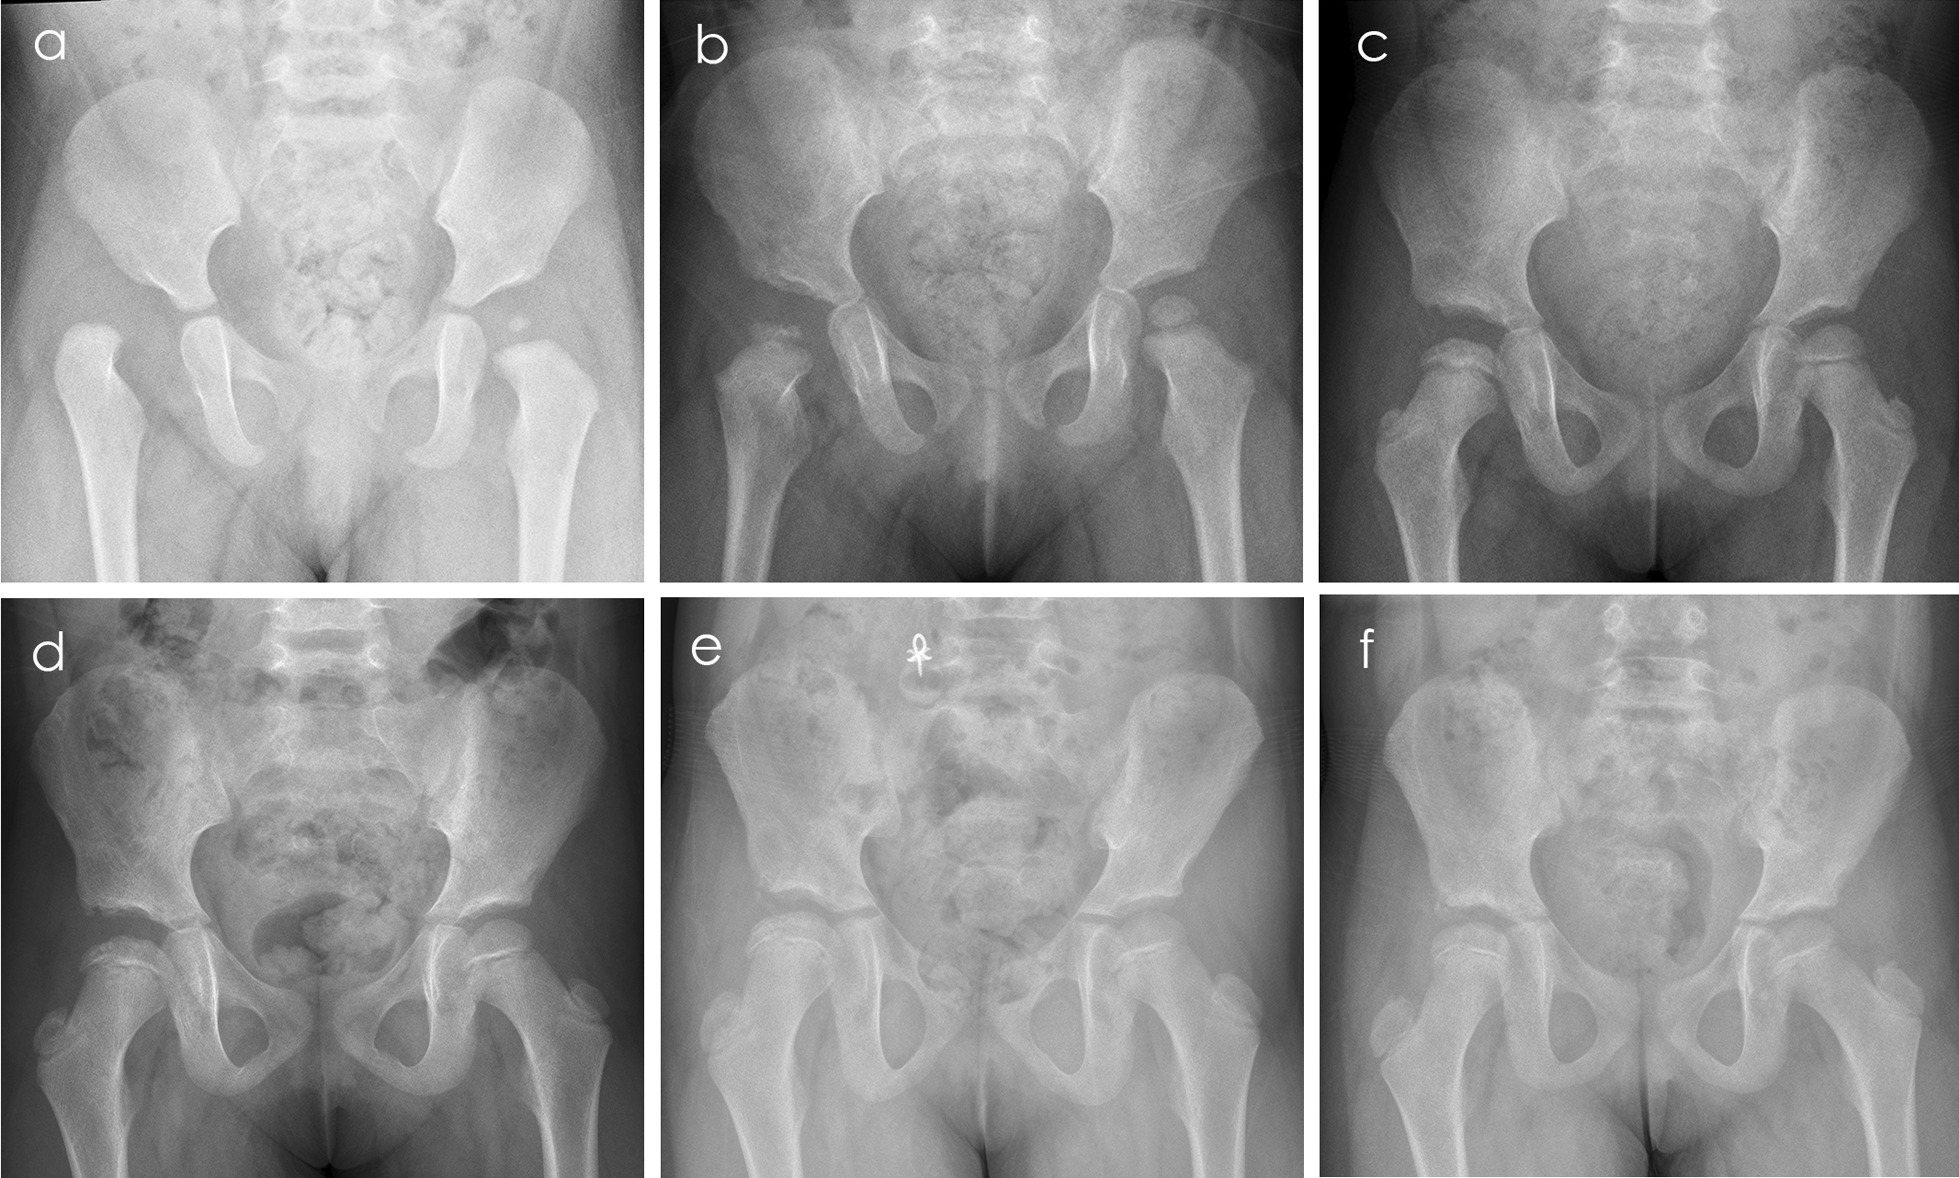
a Preoperative pelvic anteroposterior view in a 15-month-old girl on right hip dislocation with the classical Smith–Petersen approach. b–f Radiograph at 10, 40, 54, 75, and 87 months after open reduction, respectively, which shows Type 2 AVN
